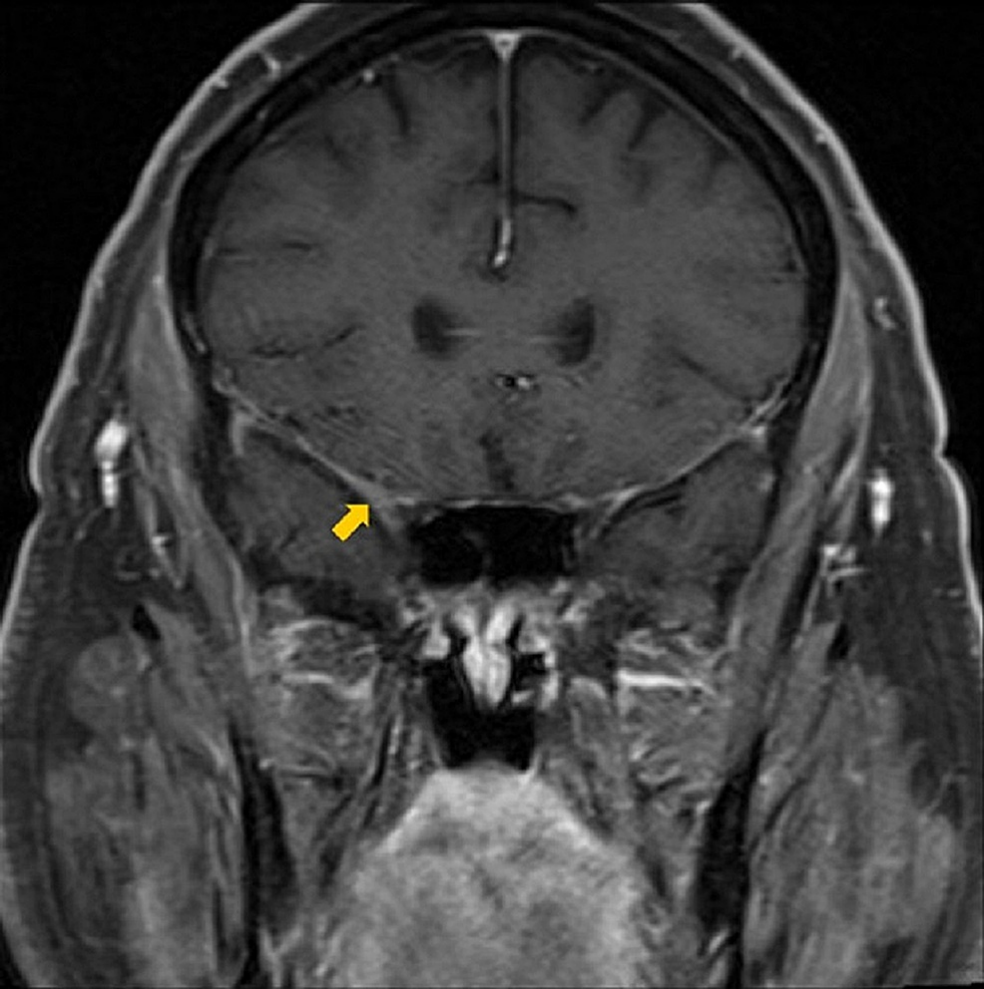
Three-month follow-up coronal contrast-enhanced T1-weighted MRI The image is showing markedly decreased inflammation of the right posterior orbital apex and right cavernous sinus (yellow arrows) after treatment with prednisone 60 mg.
